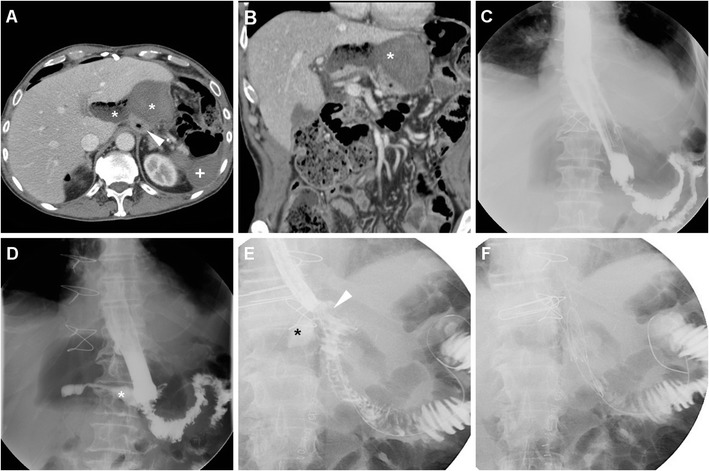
A 68-year-old male with recurrent gastric carcinoma on previous Billroth II gastrectomy for peptic ulcer was treated with total gastrectomy. Postoperative anemization required blood transfusions. On 12th POD, contrast-enhanced CT (A, B) showed persistent intraperitoneal air, a mixed attenuation collection (*) adjacent to the EJS (arrowheads), consistent with anastomotic dehiscence, a subacute blood collection (+) in the site of splenectomy. After percutaneous drainage, AL was treated with positioning of a metallic stent (C) through the EJS. Persistent leakage of oral iodinated contrast (*) at follow-up CF (D, E) led to removal of the stent and positioning of a self-expanding stent (F)
